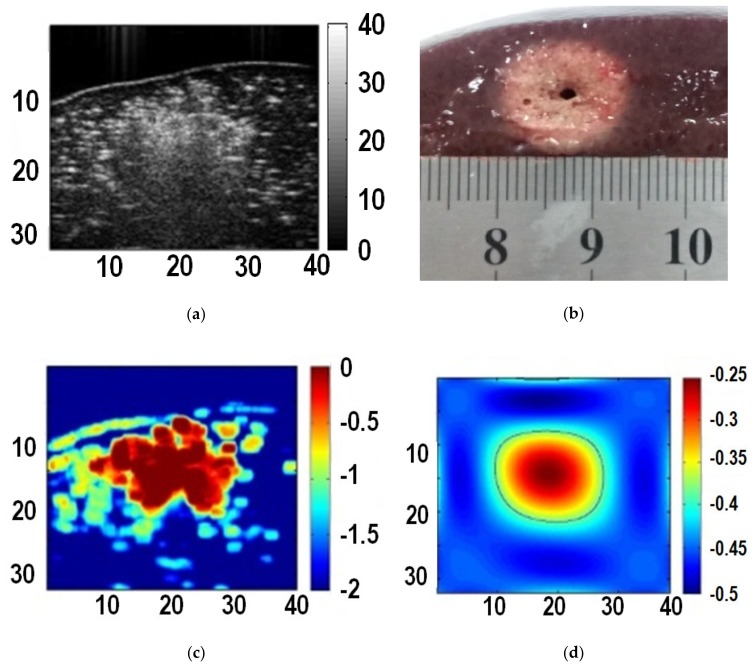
Images of liver tissue at the end of ablation. (a) B-mode ultrasound image; (b) gross pathology image of the thermal lesion; (c) the cumulative echo decorrelation image; (d) the polynomial approximation image.
